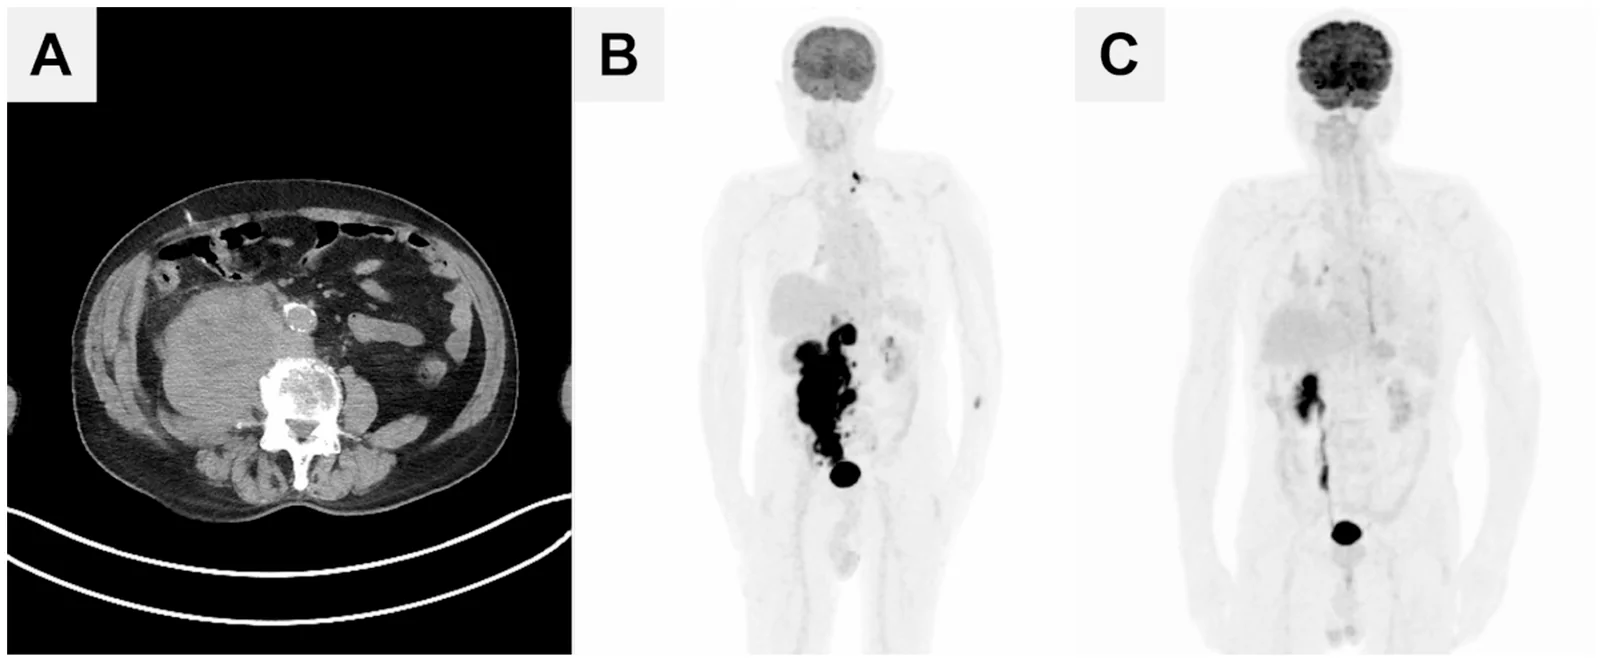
Axial non-contrast computed tomography (CT) imaging reveals a bulky psoas muscle tumor at the time of first relapse (A). 18F-fluorodeoxyglucose positron emission tomography (18F-FDG-PET) demonstrates intense FDG uptake (SUVmax: 34.63) within the lesion (B). Following four cycles of dose-reduced Pola-R-CHP and subsequent radiotherapy, maximum intensity projection (MIP) imaging of FDG-PET shows complete resolution of the FDG-avid mass, indicating a complete metabolic response (C).
